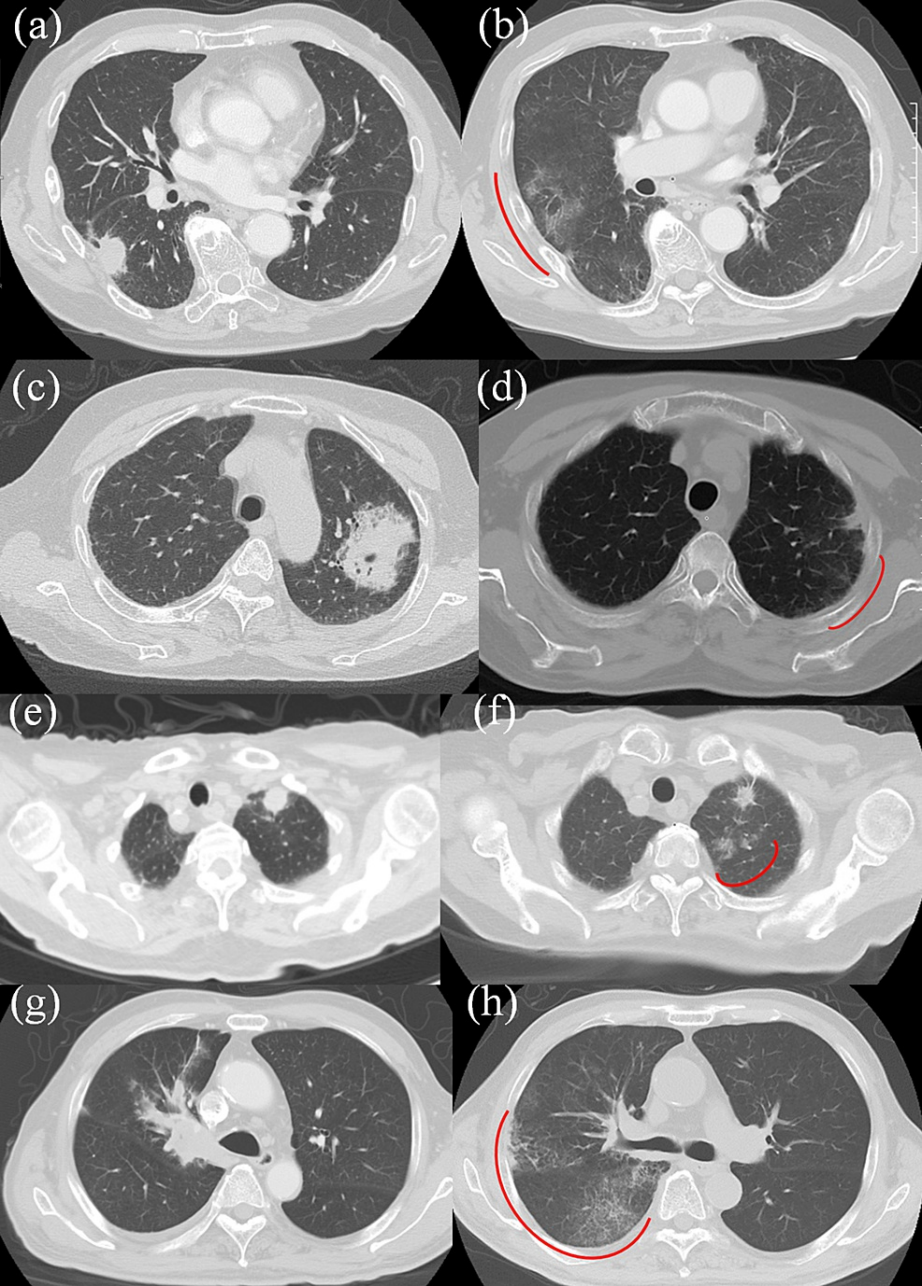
Comparison of CT images at the times of treatment planning and RP detection in cases 4 (a, b), 5 (c, d), 7 (e, f), and 8 (g, h), respectively RPs are indicated with red lines. RP - radiation pneumonitis
